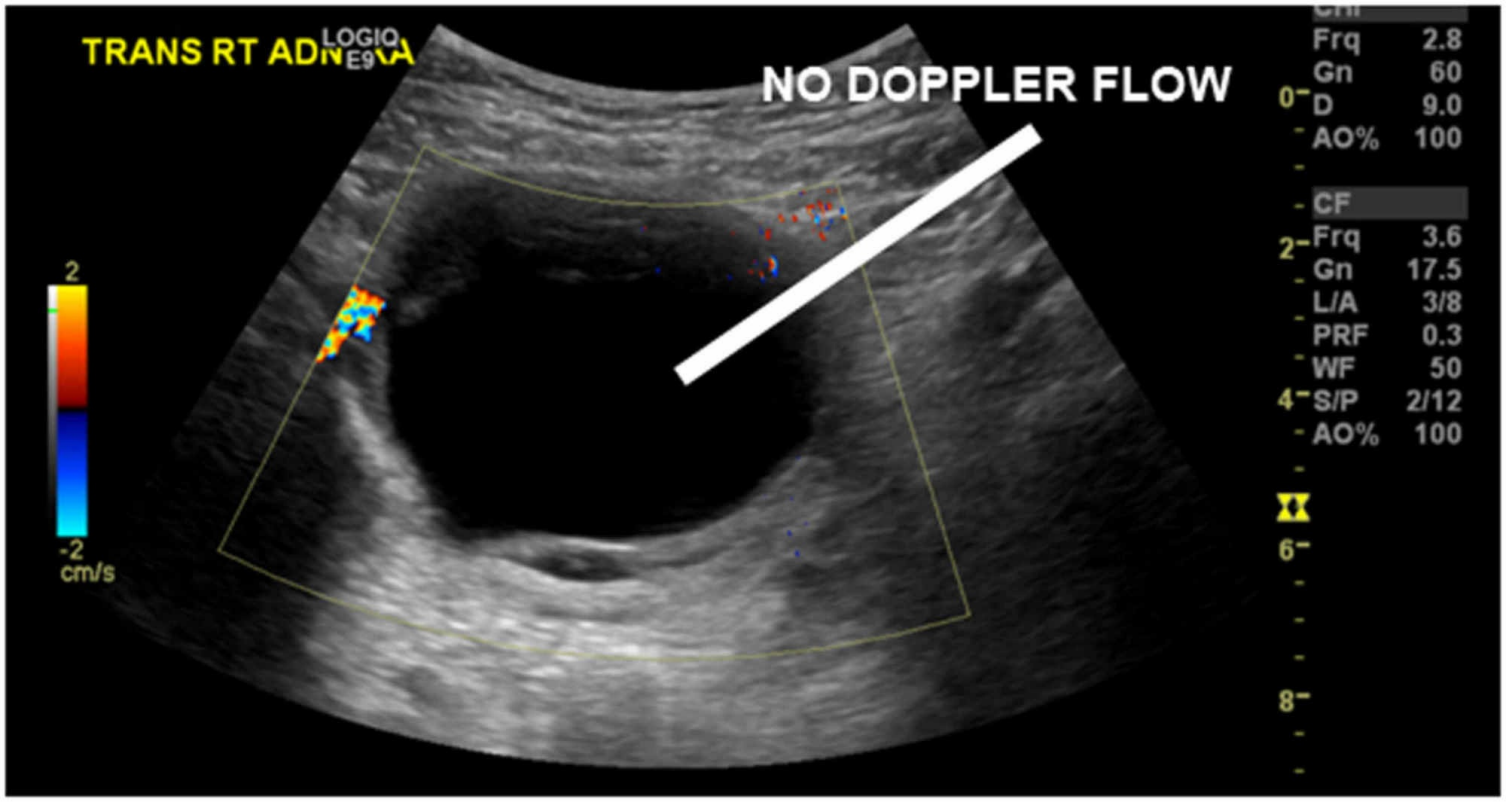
Trans-abdominal pelvic ultrasound (right adnexa with Doppler)
